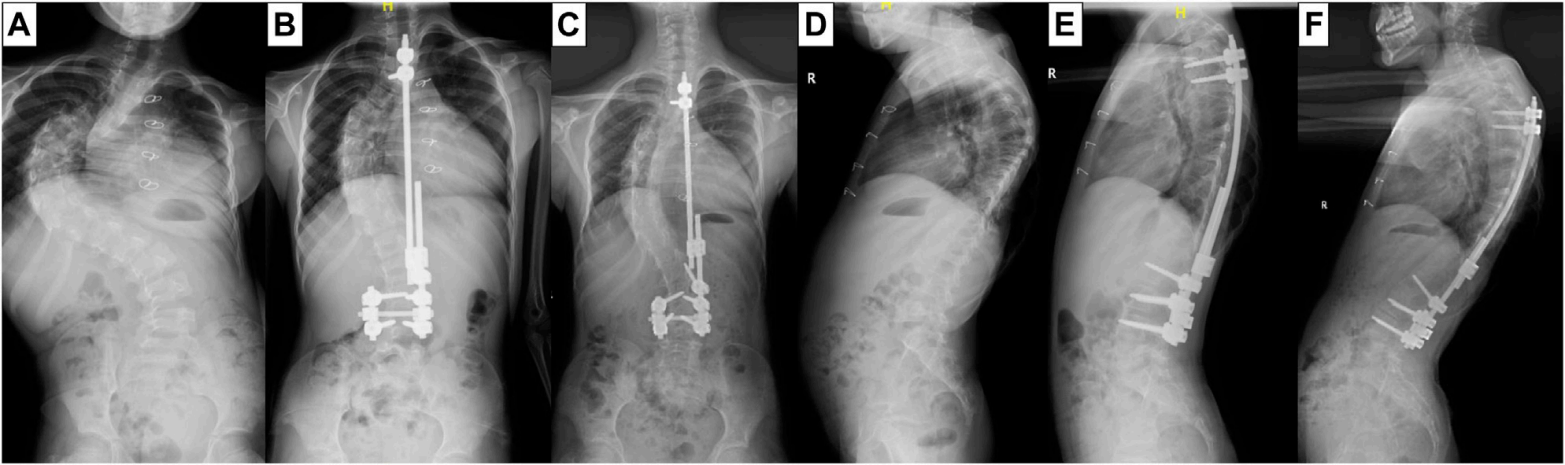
X-ray film of the patient before GR surgery (A,D), after surgery (B,E) and at the follow-up (C,F).
